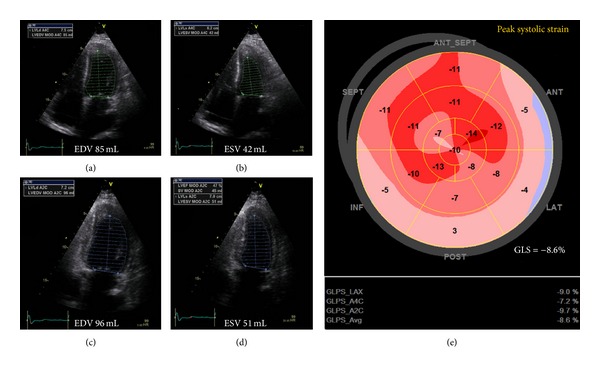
Echocardiography 29.12.2010. (a) and (b) End-diastolic and end-systolic apical 4-camper view. (b) and (c) End-diastolic and end-systolic apical 2-camper view. LV-EF 49%. (e) Bulls plot of global longitudinal strain = −8.6%.
